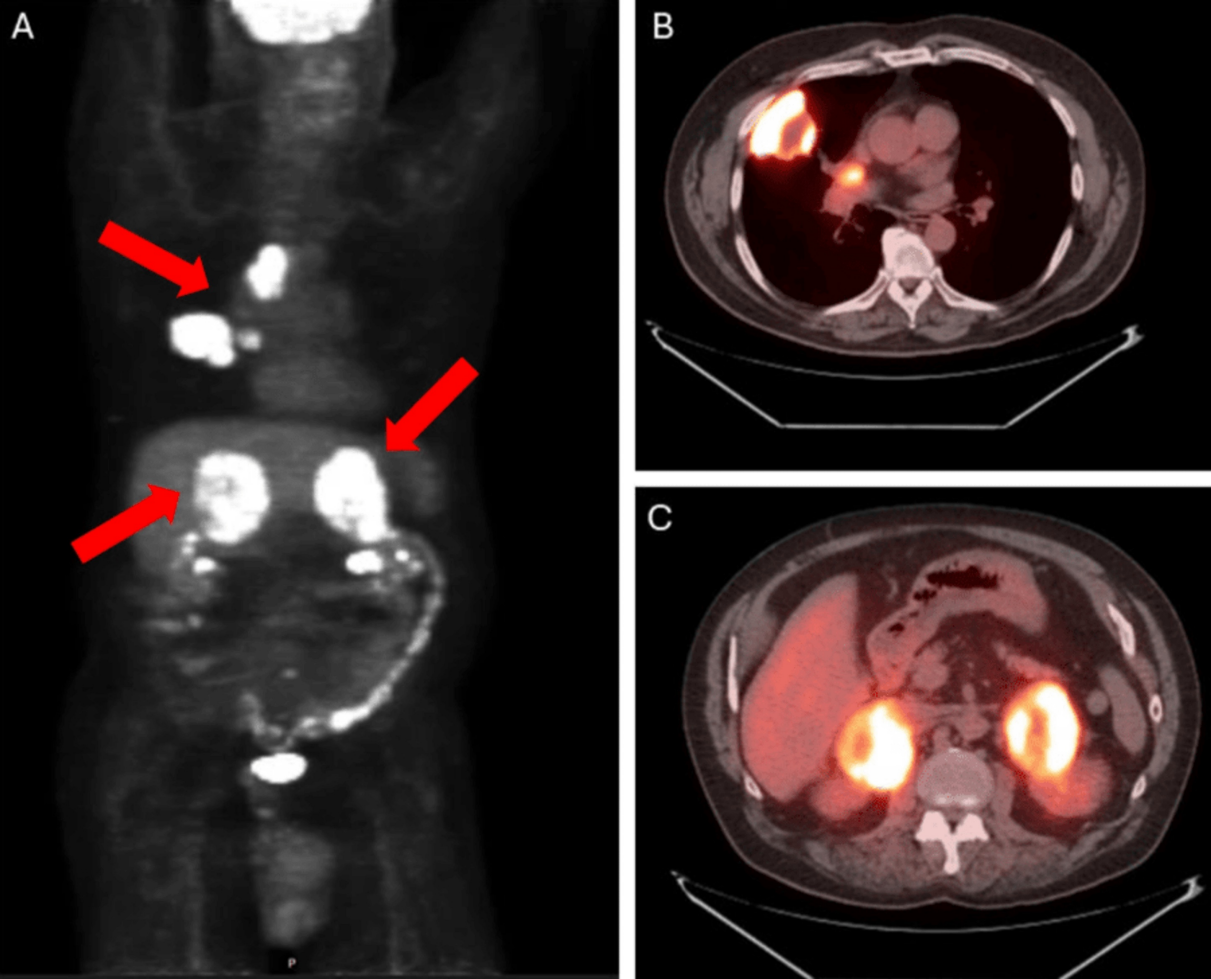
Initial staging PET-CT demonstrated multiple sites of FDG-avid disease. (A) Skull base to mid-thigh PET-CT images with arrows indicating thoracic and bilateral adrenal disease; (B) axial slice demonstrating lung mass and mediastinal disease; (C) axial slice demonstrating bulky bilateral adrenal metastases.
